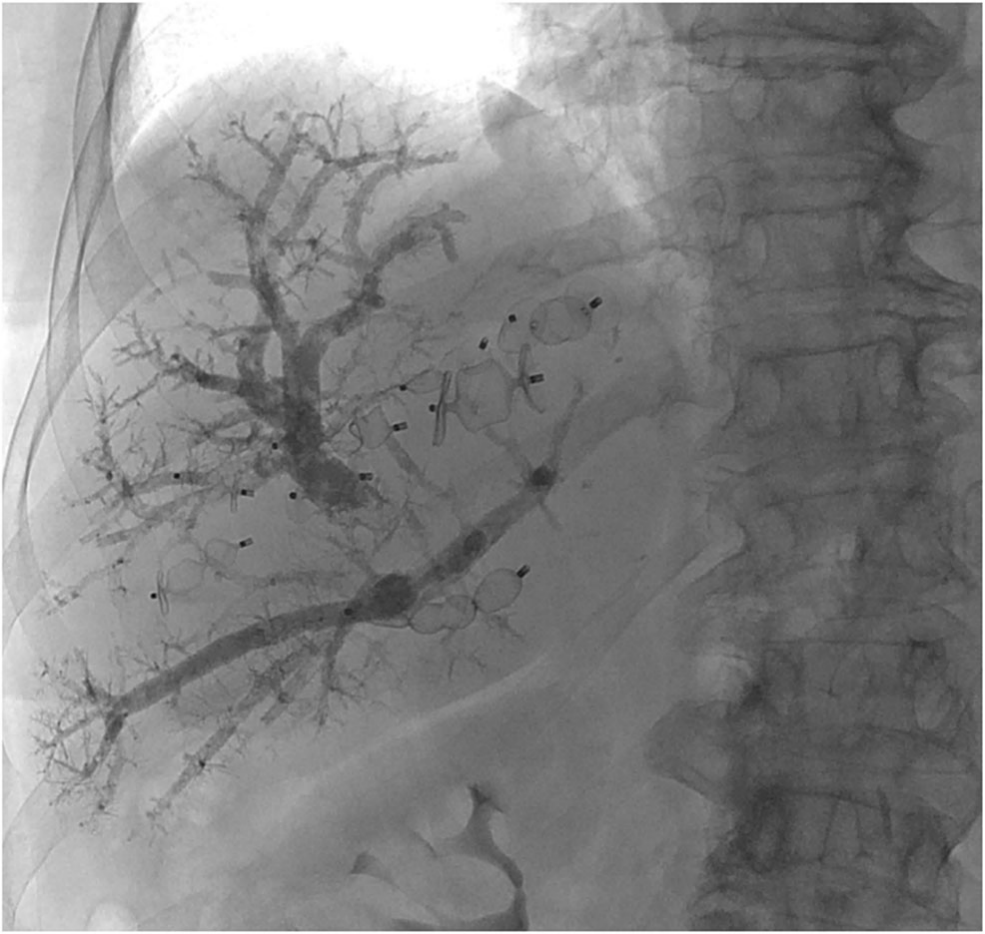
CT scan of a patient using multiple AVPs for right-sided hepatic vein embolization
